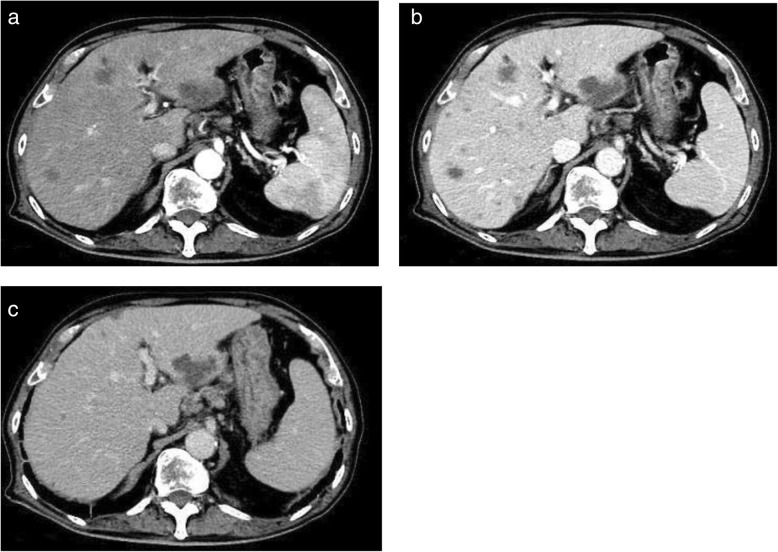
Contrast-enhanced computed tomography image of the abdomen showing multiple hypovascular nodules in the liver, spleen, and para-aortic lesions. a Arterial phase, b portal vein phase, c late phase
